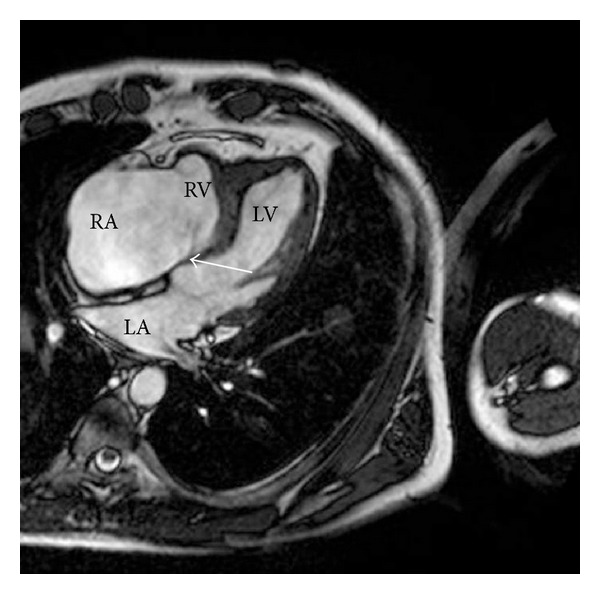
Cardiac MRI shows dilatation of the right atrium and severe tricuspid regurgitation. Small right ventricular cavity and slightly displaced offset of the tricuspid valve are seen, characteristics for Ebstein's anomaly (arrow). RA: right atrium; RV: right ventricle; LA: left atrium; LV: left ventricle.
